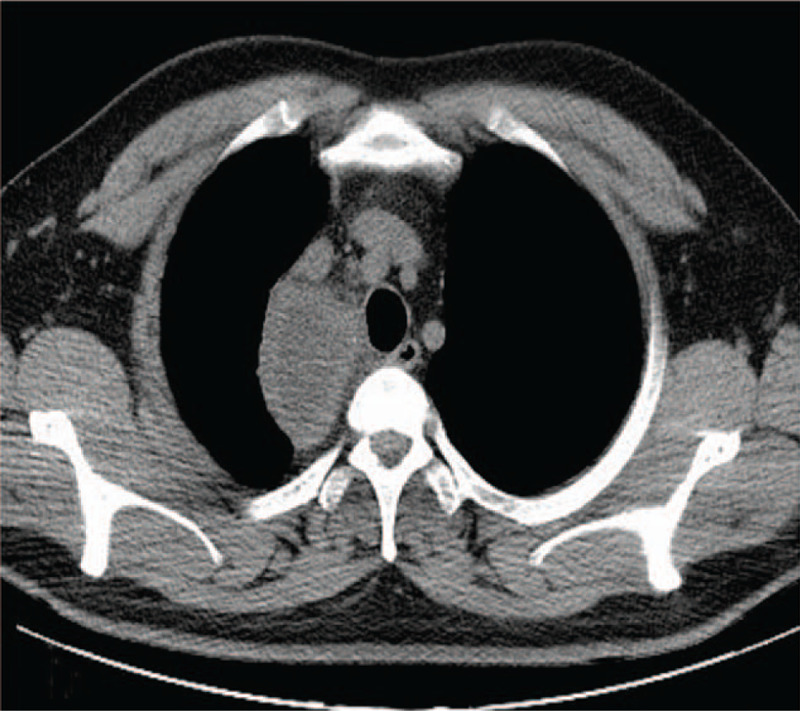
Chest CT on July 17 showed the cyst was smaller than the size of pre-EBUS-TBNA with a thicker wall.
